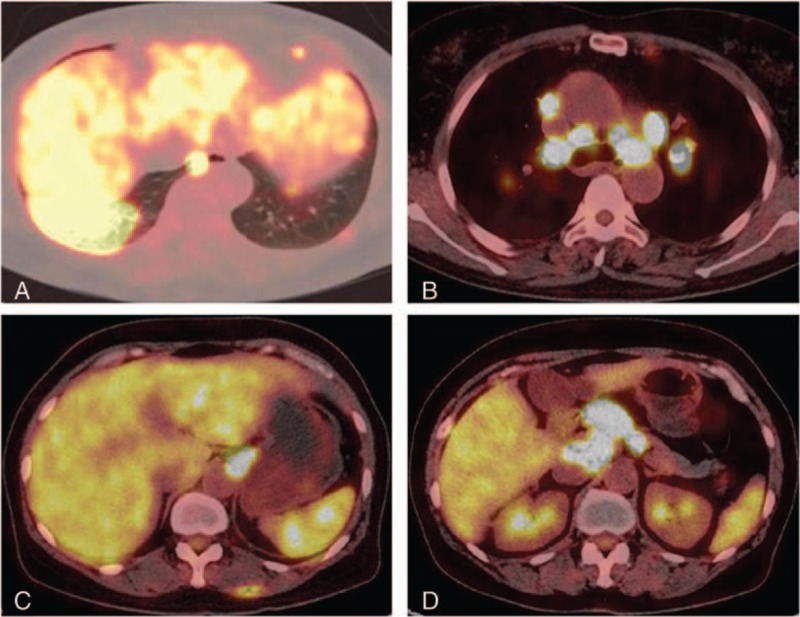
Selected transaxial F-18FDG PET/CT slices of high uptake of F-18 FDG in the mass in the lower lobe of the right lung (SUV_max_ = 20.44), bilateral pulmonary hilar and mediastinal lymph nodes (30.4), and around the lymph nodes near the pancreatic head (43.58). F-18 FDG PET/CT = ^18^F-fluorodeoxyglucose positron emission tomography/computed tomography, SUV_max_ = maximum standardized uptake.
